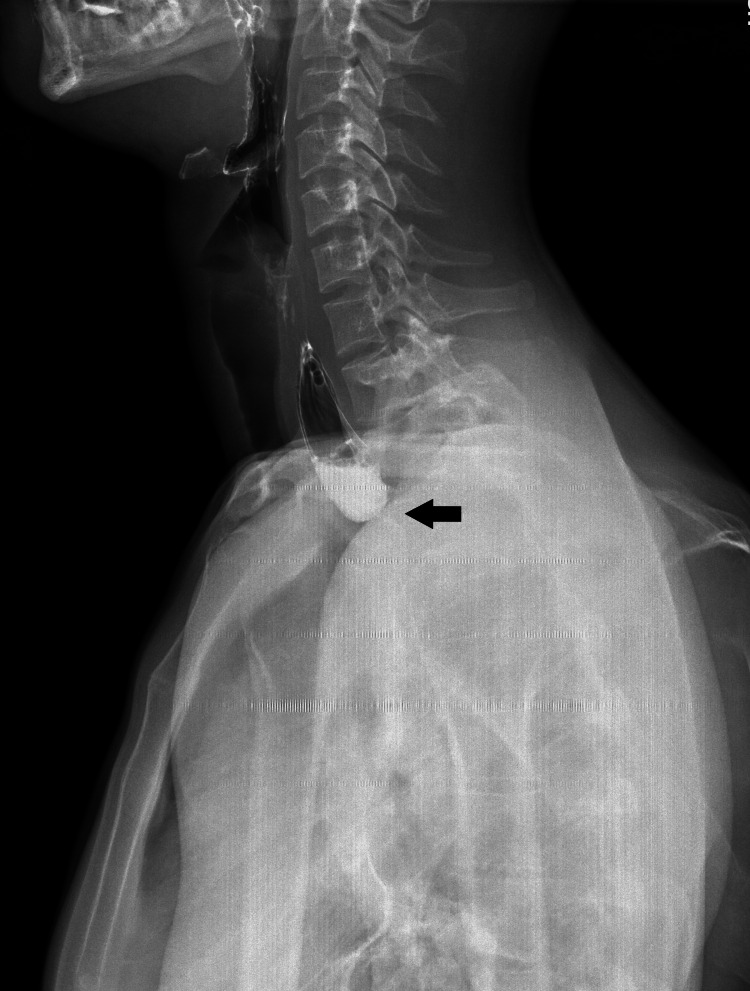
Contrast X-ray after Ivor-Lewis gastroesophagoplasty A visualization of the contrast medium stop at the transition between the neck and chest just above the previously performed Ivor-Lewis esophagogastric anastomosis and the localization of the stricture itself (black arrow)
